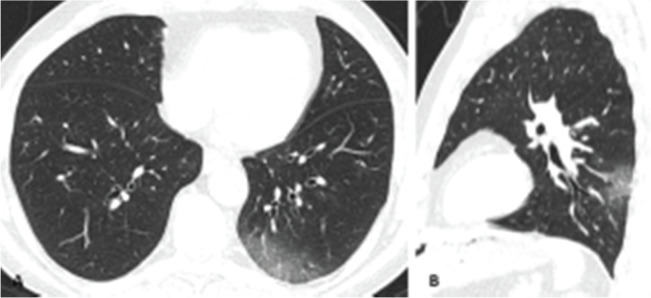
Indeterminate findings of COVID-19. Axial (A) and sagittal (B) CT images show unilateral GGOs in the upper segment of the left lower lobe.
